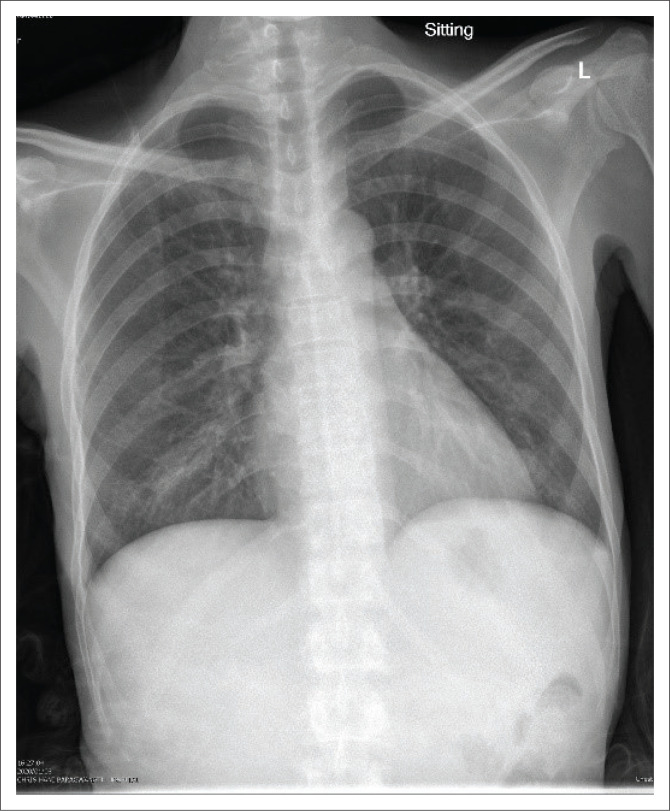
Chest radiograph on patient on readmission (1-month post-antituberculosis treatment). Patchy consolidation of the right upper lobe, as well as the right and left lower lobes. Silhouetting of the right heart border. These are features in keeping with bronchopneumonia with right middle lobe consolidation and/or atelectasis.
